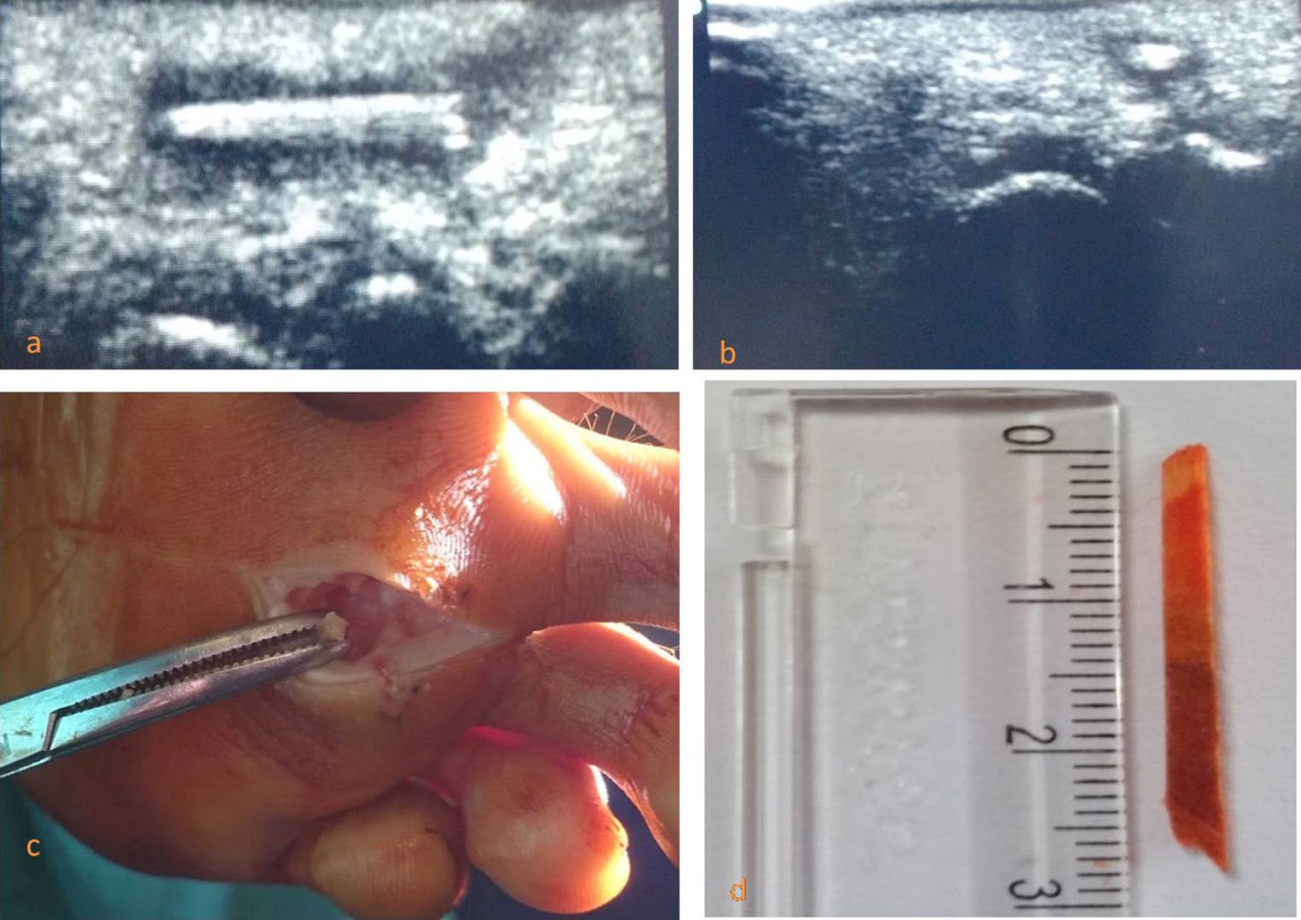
Ultrasound of foot of the patient with chronic discharging sinus (a & b). Surgical removal (c) and wood foreign body (d)
